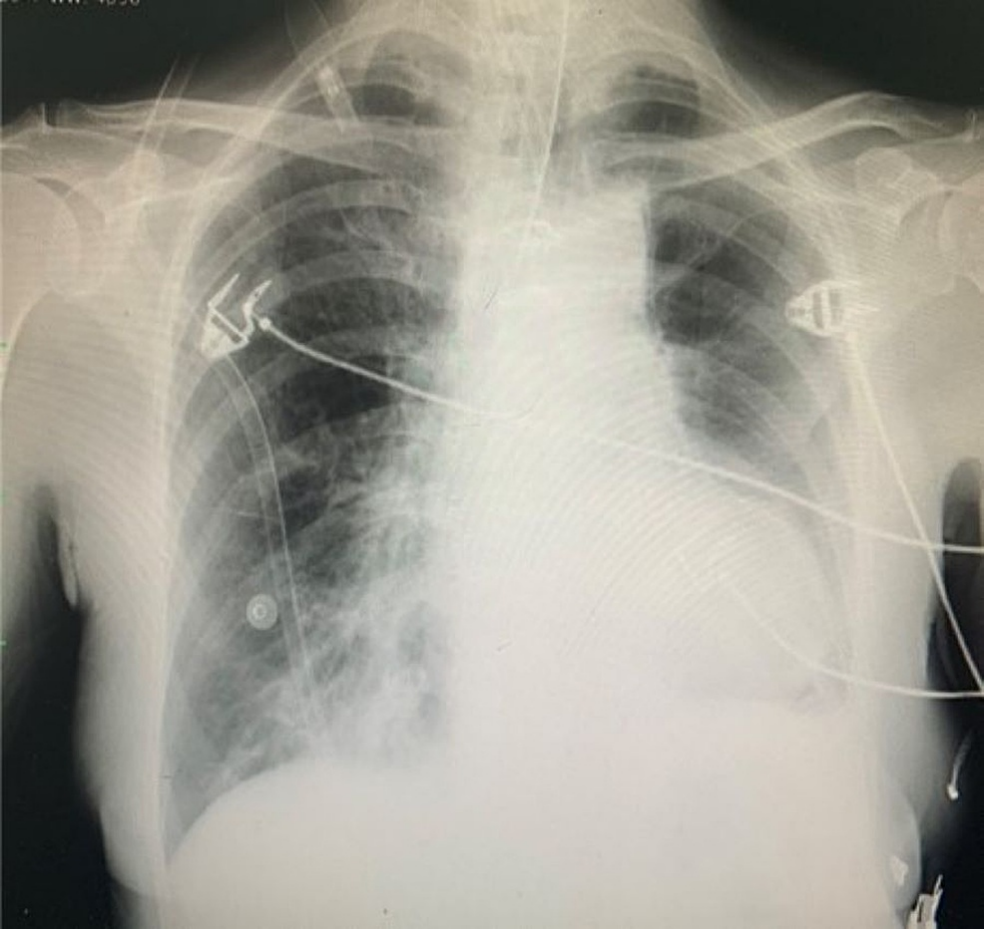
Post-intubation chest x-ray
